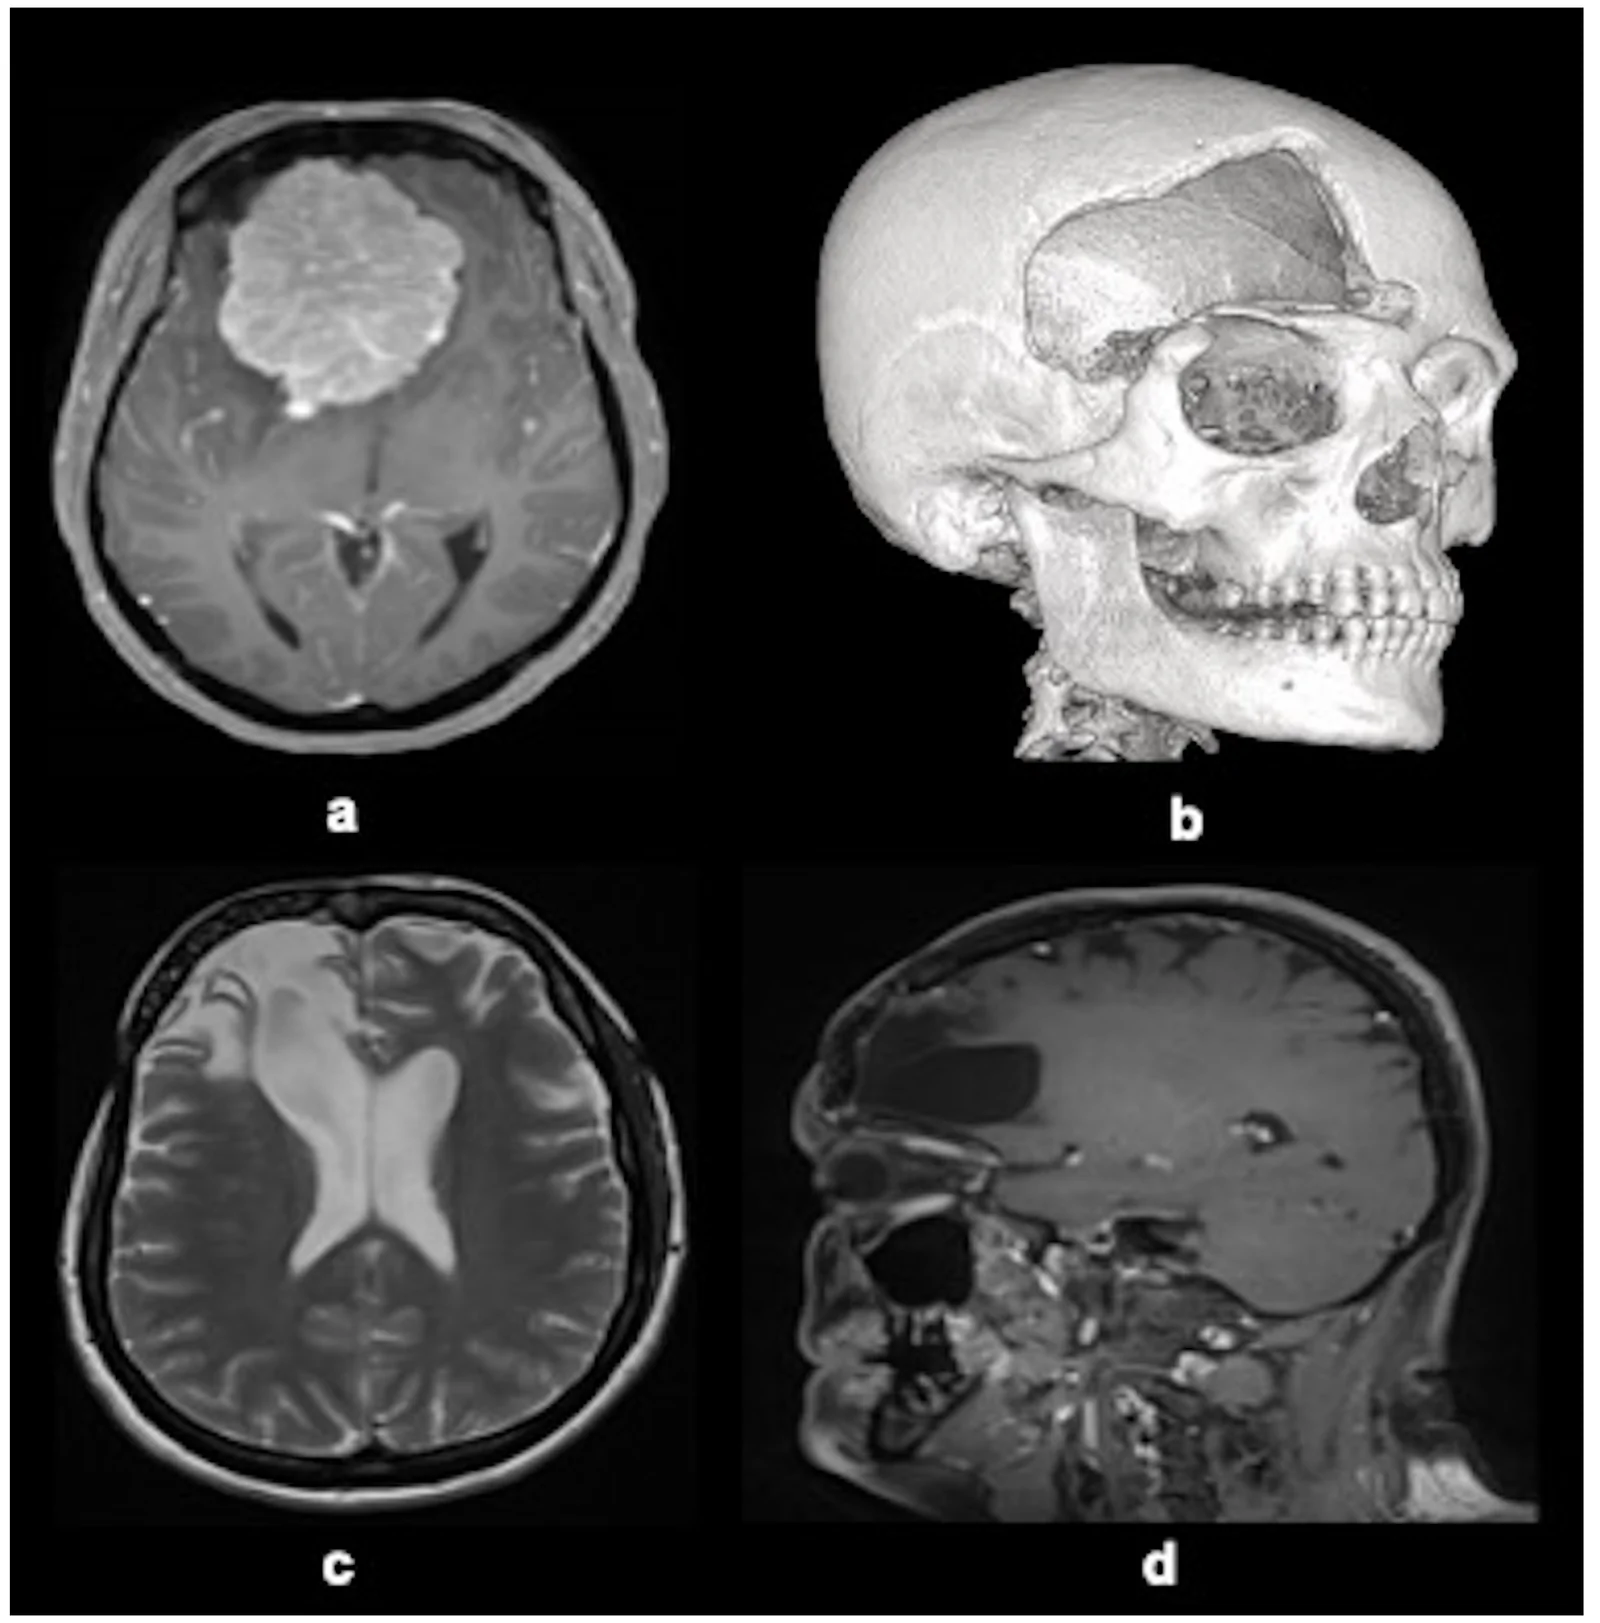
Case example MR images. (a) preoperative axial T1-weighted with gadolinium showing a large olfactory groove meningioma. (b) postoperative CT scan with 3D reconstruction showing a large aesthetic defect in the right frontal region. (c) Postoperative axial T2 weighted and (d) T1 weighted sagittal images with gadolinium showing the prosthesis fitting the cranial defect without imaging artifact.
